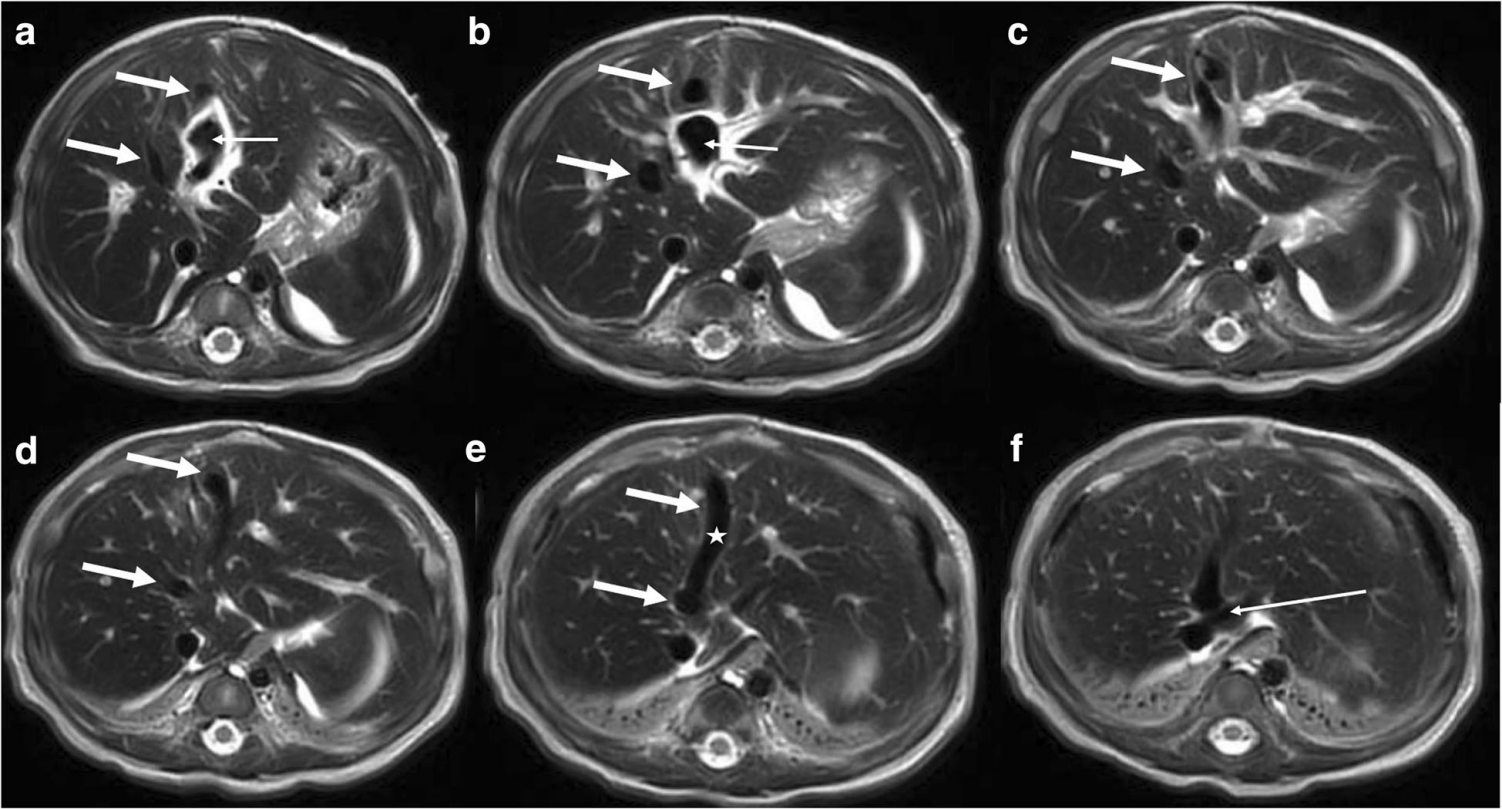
Series of abdominal axial magnetic resonance images showing the two intrahepatic shunts. a–f Caudal to cranial. The two shunts (marked by *thick white arrow*) originate from the recessus umbilicalis (marked by *thin white arrow* in a and b) of the left portal branch, draining into the dilated medial liver vein (*white star* in e) and then into the vena cava inferior (*white thin arrow* in f)
